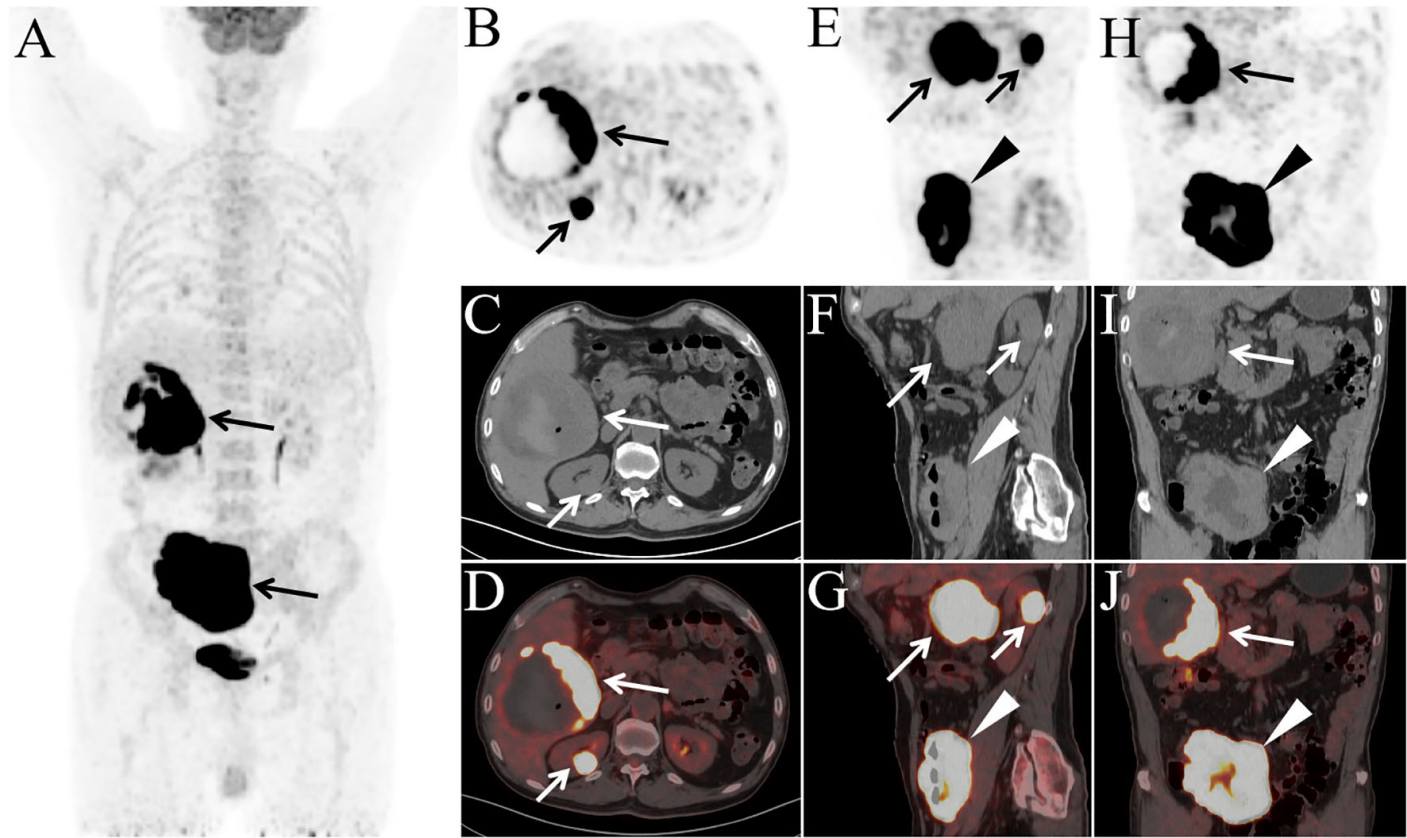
The patient underwent PET/CT examination for staging [(A) MIP; (B, E, H) PET; (C, F, I) CT; (D, G, J) PET/CT]. MIP image shows abnormally increased FDG uptake in the right upper and lower abdominal regions (A, arrows). Axial (B–D), sagittal (E–G), and coronal (H–J) PET/CT images demonstrate a large mass in the posterior segment of the right hepatic lobe measuring 11.0 cm × 10.2 cm × 10.5 cm (B–J, long arrows), a slightly hyperdense nodule in the right kidney measuring 2.6 cm × 2.2 cm × 2.6 cm (B–G, short arrows), and diffuse thickening of the ileal wall with a maximum wall thickness of 4.3 cm (E–J, arrowheads), all exhibiting markedly elevated FDG uptake (SUVmax: hepatic lesion, 32.3; renal lesion, 34.1; ileal lesion, 34.3). Central necrosis, hemorrhage, and a small amount of intralesional gas were observed within the hepatic mass. Notably, there was no evidence of lymphadenopathy or abnormal FDG uptake in any lymph nodes throughout the body.
